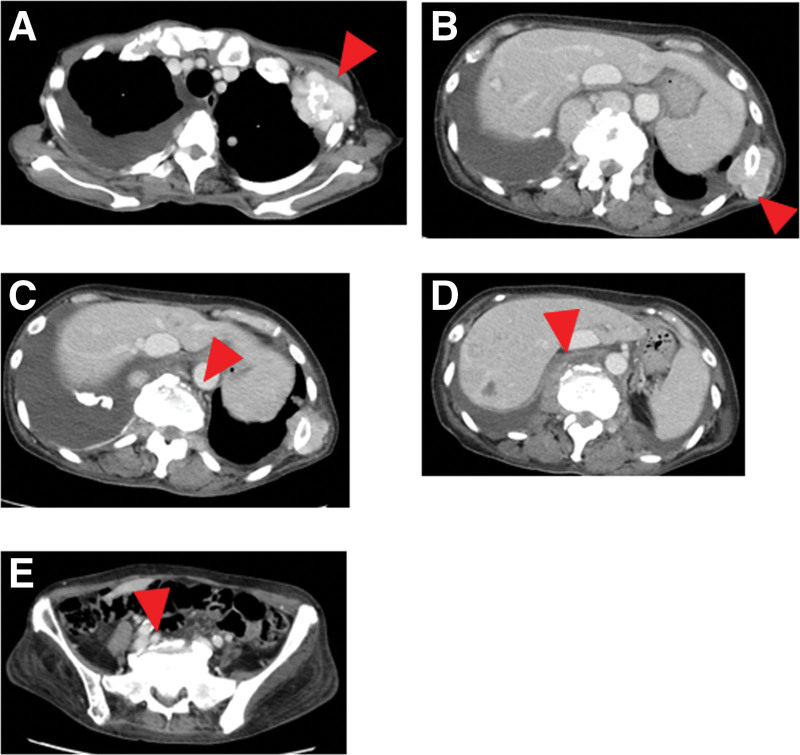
CT axial images reveal the metastatic lesion in the second rib (A, red arrowhead), tenth rib (B, red arrowhead), and multiple spinal metastatic lesions (C–E, red arrowheads).
